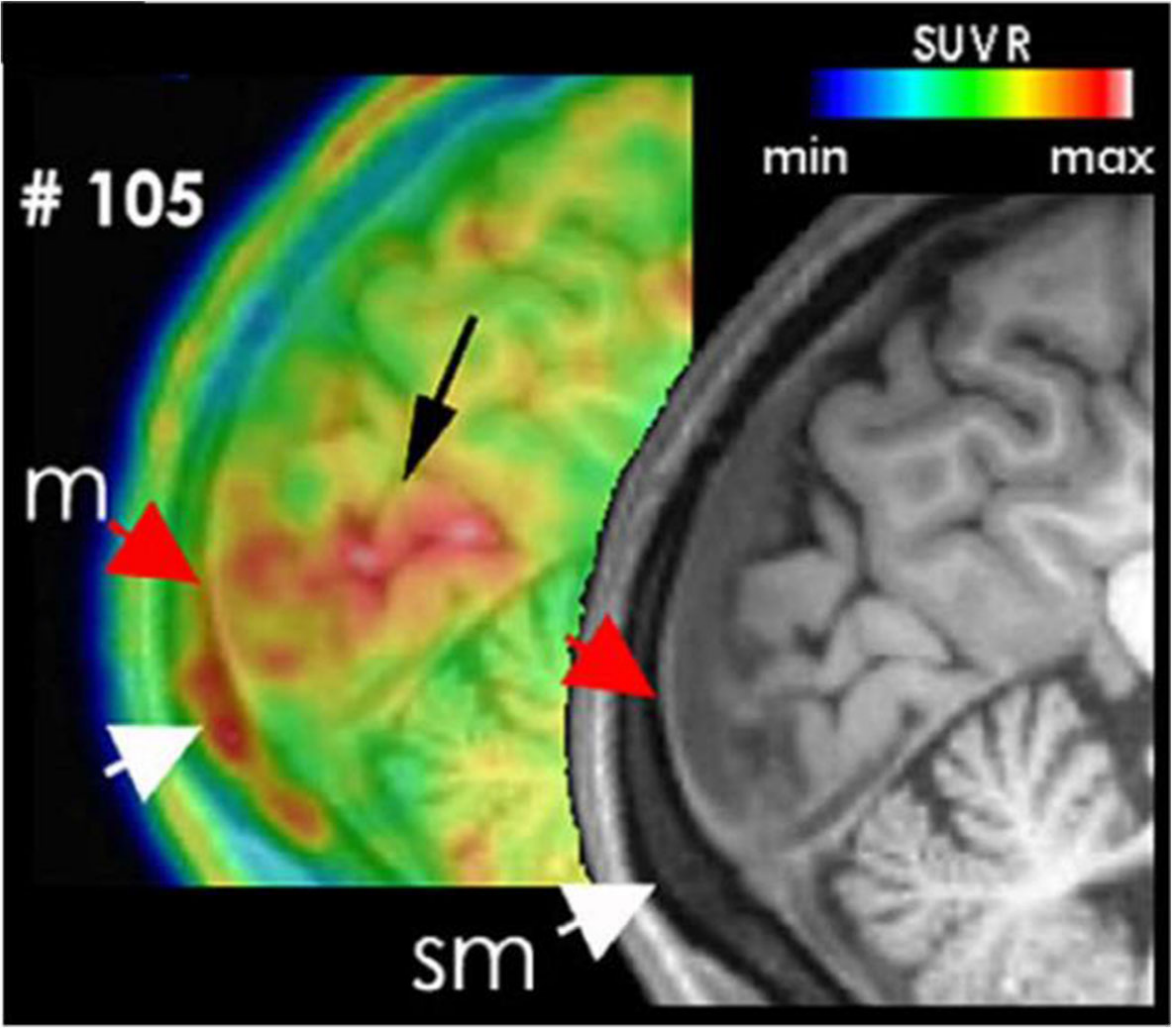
[(11) C]PBR28 PET/MRI showing inflammatory activity in the occipital cortex (black arrow), overlying meninges (red arrows), and bone marrow (white arrows) in a patient suffering from migraine with visual aura attacks (from [13] with permission)
